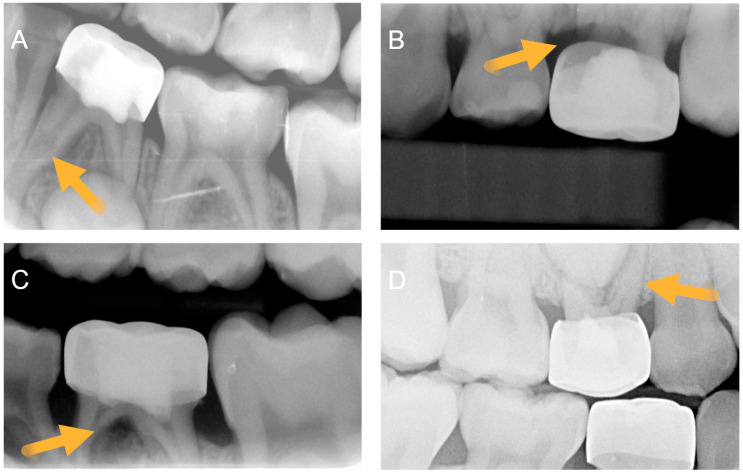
Post-pulpotomy radiographic assessment of (A) internal root resorption (IRR), (B) perforating IRR (IRR_P), and (C) furcal radiolucency (FRL). RR, IRR_P, and FRL were defined as signs of radiographic pathosis. (D) assessment of pulp canal calcification (PCC) that was defined as a non-pathological process. Arrows highlight representative conditions described.
